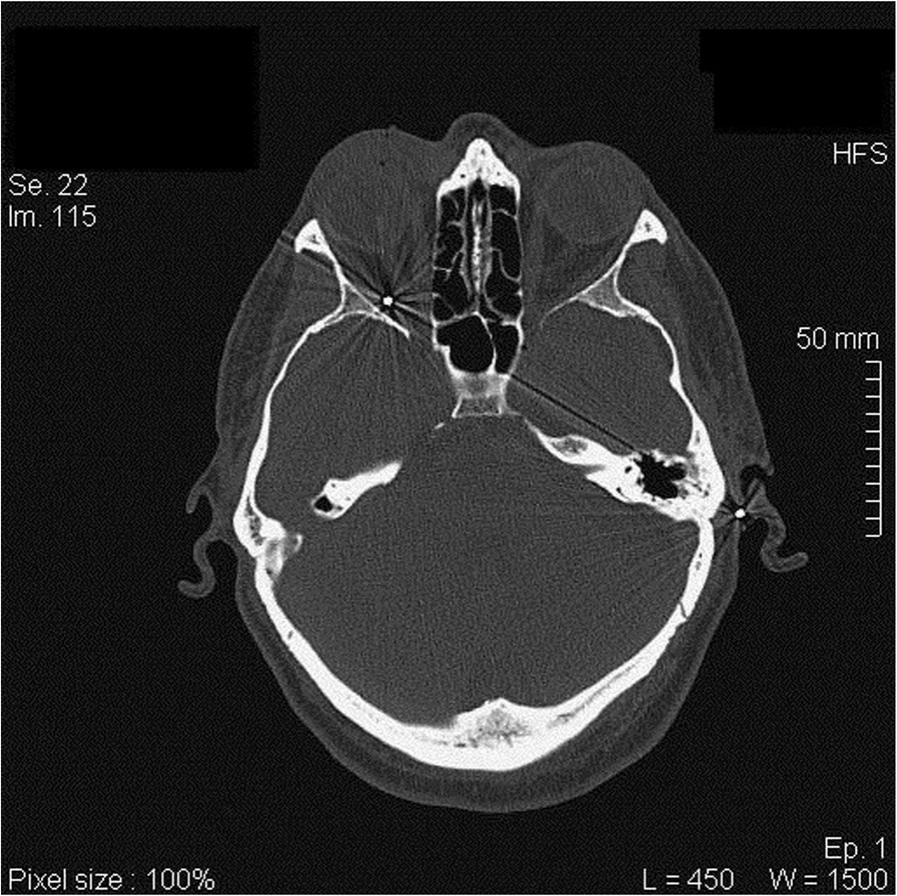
CT scan Axial Reconstruction: identification and localization of the intraorbital lead pellet
